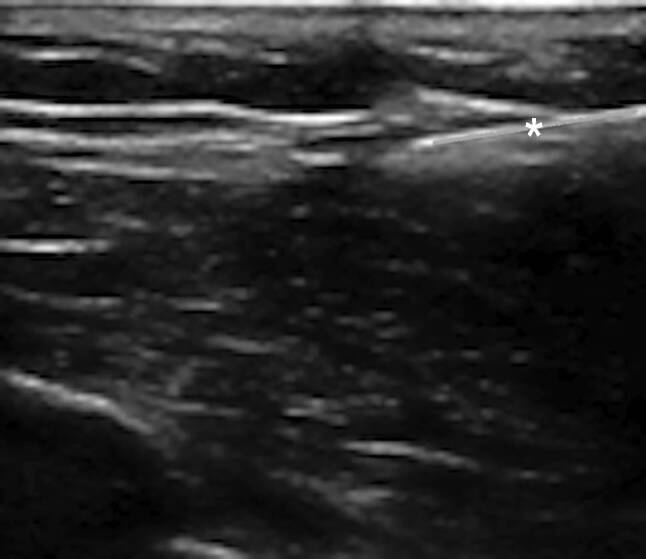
Ultrasound of the soft tissue overlying the chest and the vascular access site. Local anesthetic is injected into the prepectoralis fascia to enable a rapid anesthetic effect (*)
